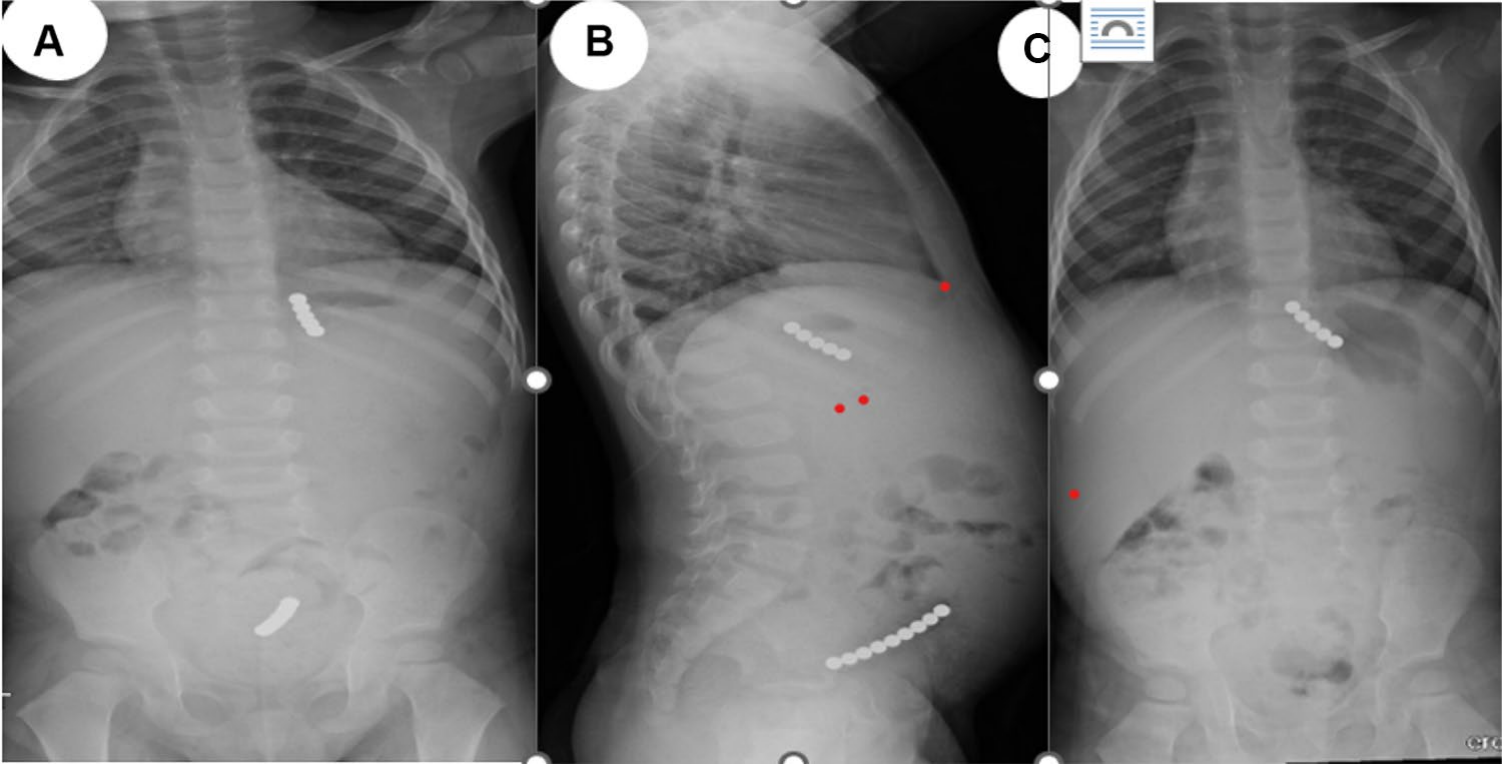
Radiograph showing multiple magnets (A) AP view; (B) Lateral view; (C) lower set of magnets passed after fleet enema and upper set removed endoscopically.
